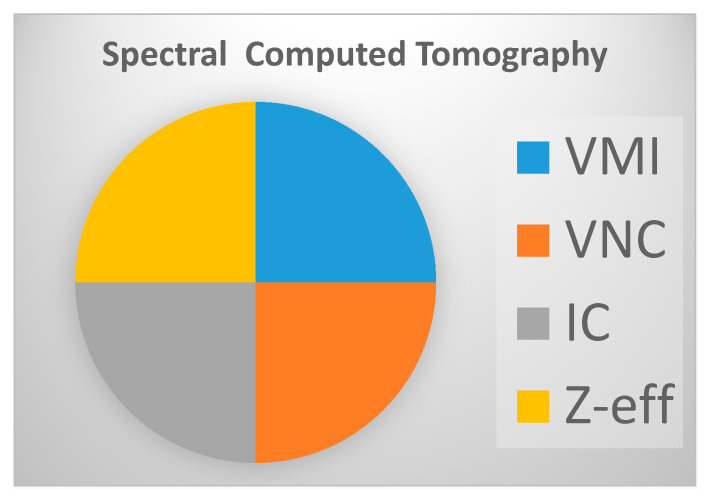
Imaging possibilities in Dual Energy Computed Tomography (DECT). VMI—virtual monochromatic images; VNC—virtual non contrast; IC—iodine concentration; Z-eff—analysis based on effective atomic number.
